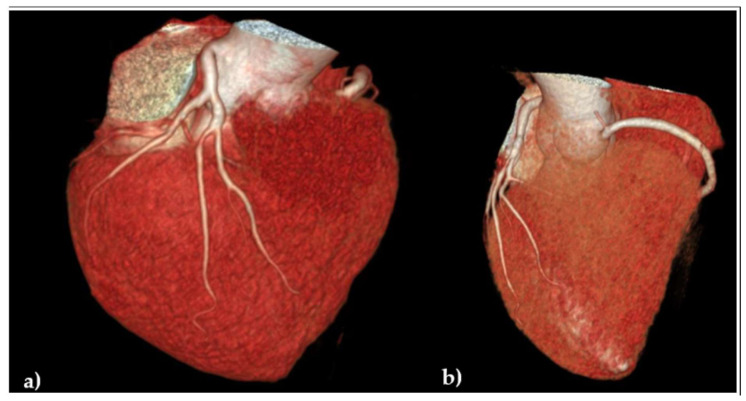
Angio-coronaro-computed tomography: volumetric reconstruction of coronary arteries: (a) frontal anterior view evidencing the left coronary artery without significant stenosis; (b) transversal cranial view revealing the origin of the right coronary artery.
